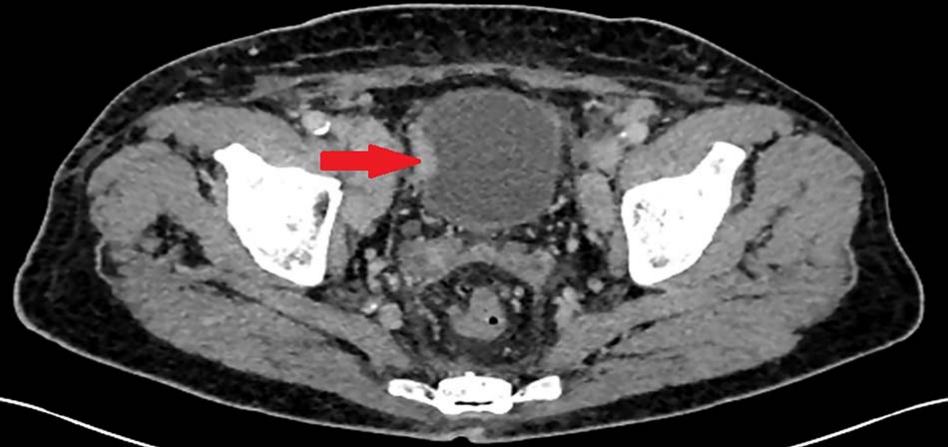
Thickening of the right lateral wall of the bladder enhancing at arterial time on CT scan (arrow).
